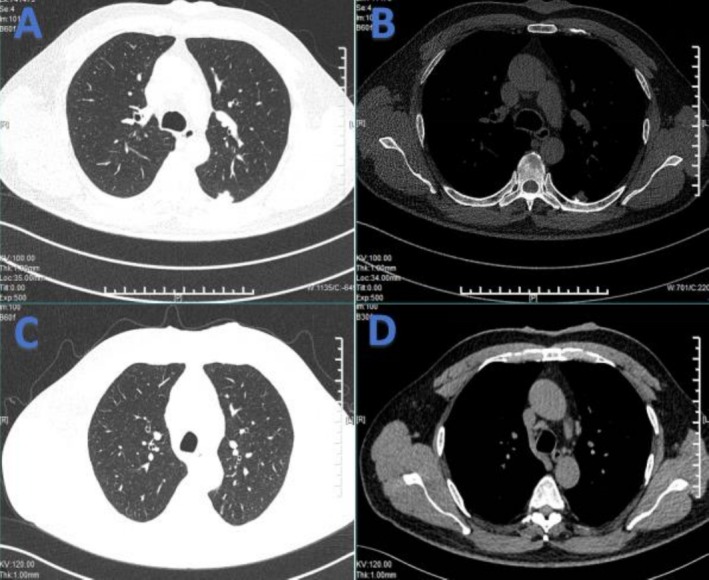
Preoperative (A, B) and postoperative (C, D) chest CT.
